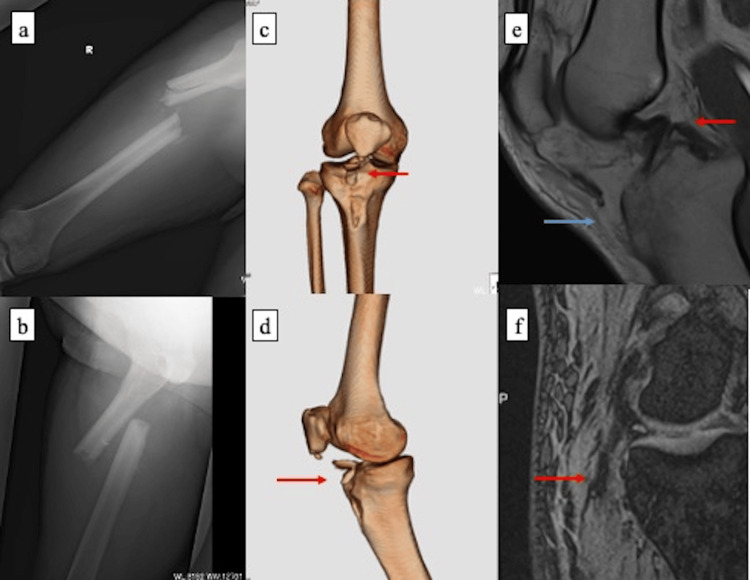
Preoperative radiographic, CT, and MRI findings (a-b) Anteroposterior and lateral radiographs of the right femur demonstrating a femoral shaft fracture. (c-d) CT images of the right knee demonstrating an avulsion fracture of the tibial tuberosity (red arrows). (e) MRI of the right knee demonstrating a posterior cruciate ligament injury (red arrow) and a tibial tuberosity avulsion fracture (blue arrow). (f) MRI demonstrating a lateral collateral ligament injury (red arrow).
